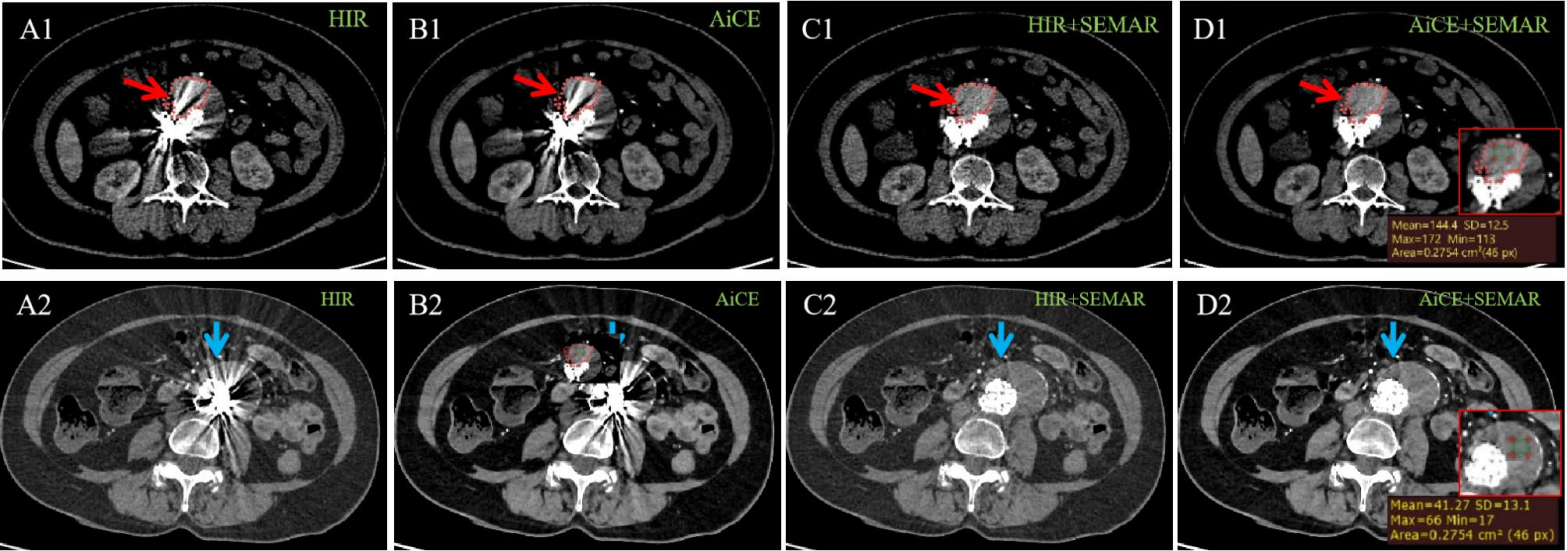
Excellent visualization of the endoleak with AiCE + SEMAR reconstruction. Axial CTA images were obtained using the HIR (A1, A2), AiCE (B1, B2), HIR + SEMAR (C1, C2), and AiCE + SEMAR (D1, D2) techniques. The images on the top row (A1-D1) represent the case of the endoleak, while the images on the bottom row (A2-D2) represent the case without the endoleak. The arrows represent the false lumen outside stents, and the red circles in the abdominal aorta (A1-D1) indicate the extent of contract leakage. The images within the red box at the bottom left corner (D1, D2), in which ROIs are put to display CT values, are the local enlarged views of the lesion areas
